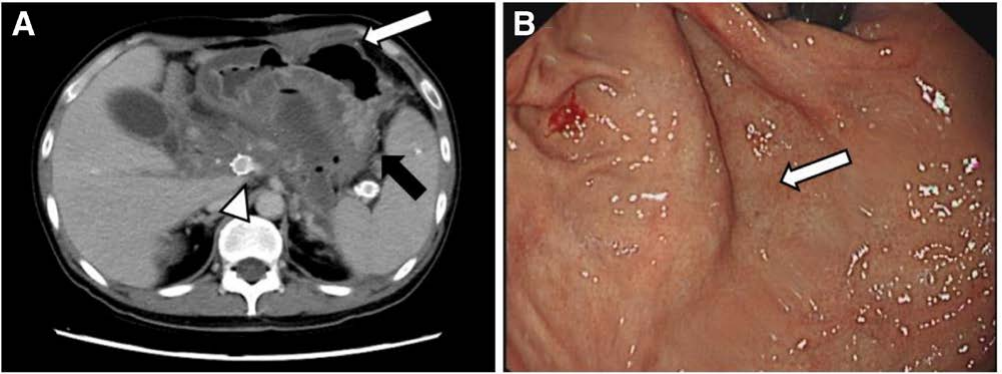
After 1 year of surgery, abdominal enhanced CT and endoscopic imaging were performed. (A) Abdominal contrast-enhanced CT showed disappearance of gastric fundus veins (black arrow) and absence of varicose gastric omentum veins (white arrow), with splenic vein stents unobstructed (arrow); (B) endoscopy shows disappearance of gastric varices (arrow).
